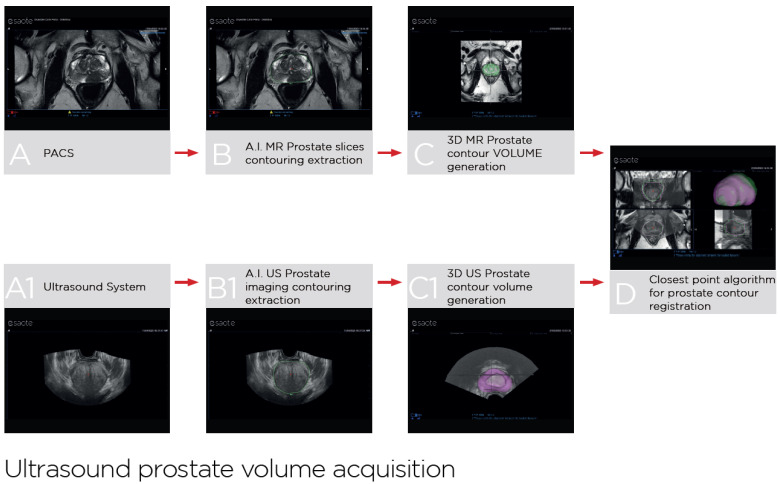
UroFusion automated prostate segmentation and fusion workflow: AI-driven automatic prostate contouring on MRI T2-weighted DICOM sequences and ultrasound volume acquired with a panoramic sweep of the gland by the probe using a specific movement transducer, leading to fused image registration. (A): T2 MRI axial view; (A1): US axial acquisition; (B): AI-driven prostate contouring on MRI; (B1): AI-driven prostate contouring on US; (C): MRI prostate contour volume generation; (C1): US prostate contour volume generation; (D): fused registration of MRI and US prostate volumes.
